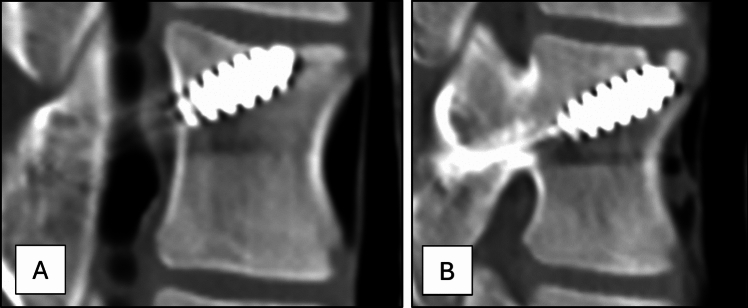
A,B Computed tomography (CT) images of vertebrae with spinal epiphysiodesis trajectory (SET) screws.A Vertebra with a SET screw that remained within the epiphysis at the end of the study.B Vertebra that “grew off” the SET screw, meaning the screw no longer crossed the epiphysis at study completion—a phenomenon previously described in screw-mediated growth modulation. The cavitation at the epiphyseal region inB suggests that the screw initially traversed the epiphysis at the time of implantation
